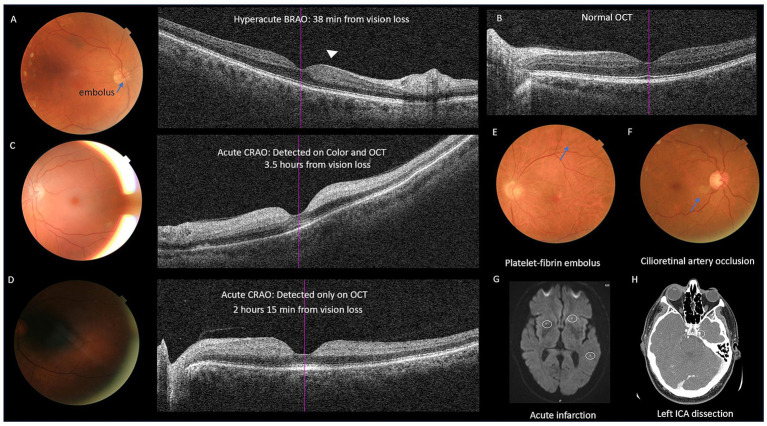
Representative cases. (A) Acute inferior BRAO in the right eye. Images were acquired 38 min after vision loss. There is a visible embolus along the inferior branch retinal artery (arrow) and no retinal whitening on the color photograph, but the OCT shows inner retinal hyperreflectivity compared to well-demarcated inner retinal layers on the OCT of the contralateral eye (B). (C) CRAO with obvious retinal ischemia with retinal whitening, cherry red spot, and box-carring of vessels on color fundus photographs and inner retinal hyperreflectivity and thickening of the ischemic retina on OCT. (D) Poor quality fundus photograph due to poor patient fixation; however, OCT shows inner retinal hyperreflectivity. (E) Large visible embolus (arrow) along the superotemporal vascular arcade. (F) Subtle cilioretinal artery occlusion (arrow). (G) Diffusion-weighted MRI showing acute small asymptomatic cerebral infarction (circled). (H) CTA head showing left internal carotid artery dissection (circled).
